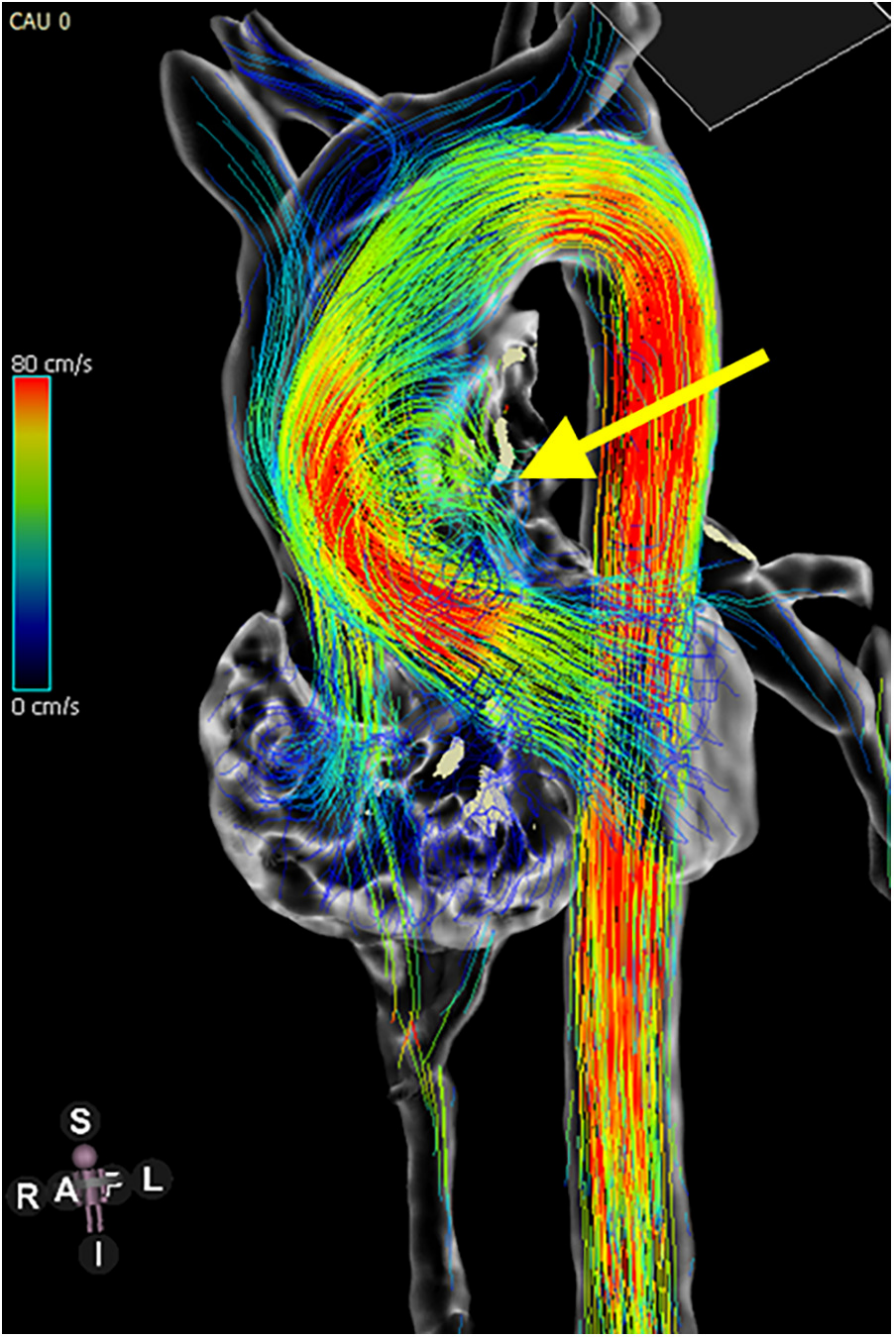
4D flow MRI showing disturbed flow in the ascending aorta of a TS patient with a bicuspid aortic valve. The yellow arrow indicates helical vortices, reflecting abnormal flow dynamics due to valve morphology.
